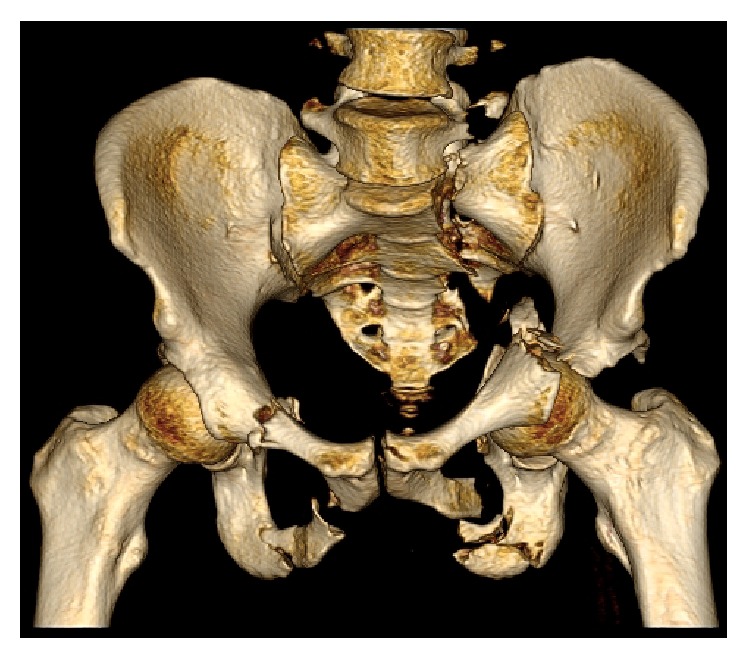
3D pelvis preoperative CT reconstruction.
